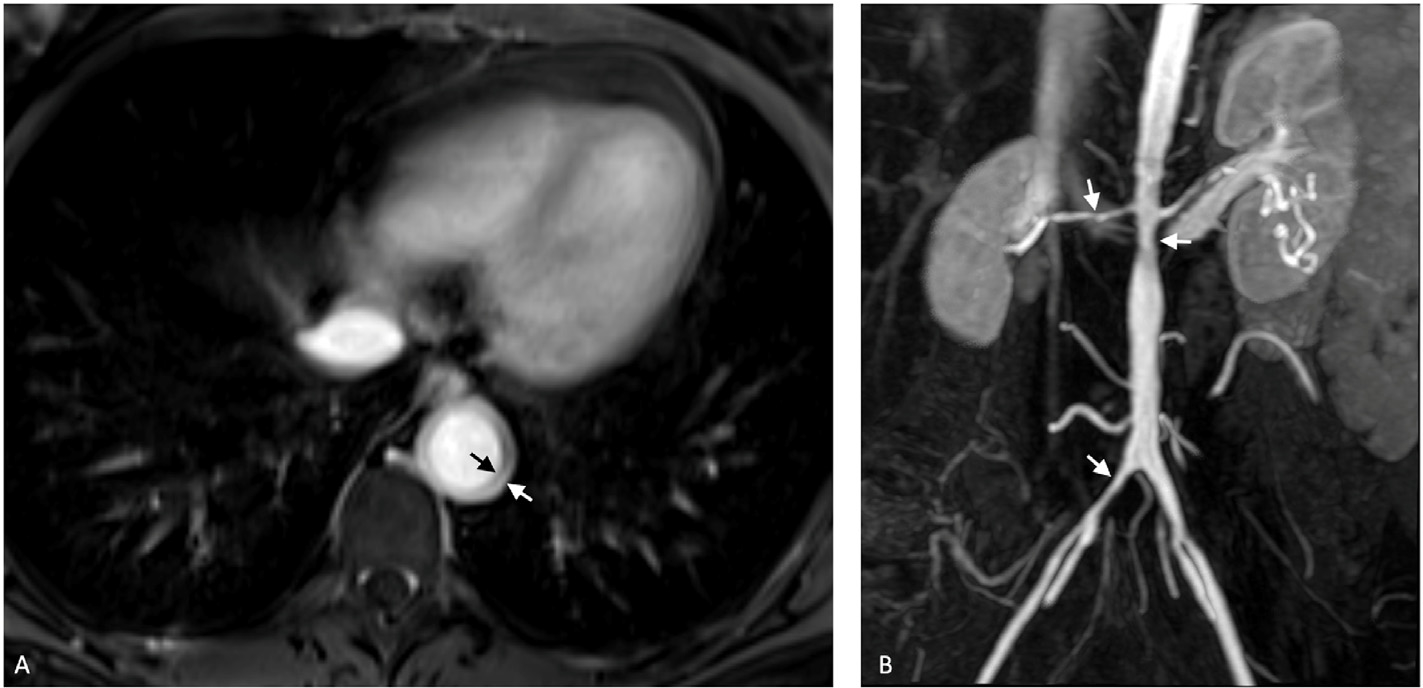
A 73-year-old female, with temporal artery biopsy-proven GCA, presented with chest pain. A postcontrast T1-weighted MRA image of the chest (A) demonstrates circumferential thickening of the descending thoracic aorta, consistent with lvGCA. A 45-year-old female with known TAK presented with hypertension. An abdominal MRA was performed. The MIP MRA image (B) of the abdomen reveals multifocal narrowing of the abdominal aorta, the right common iliac artery, and the right renal artery, as indicated by arrows. GCA, giant cell arteritis; MRA, magnetic resonance; angiography; TAK, Takayasu’s arteritis; MIP, maximum intensity projection.
